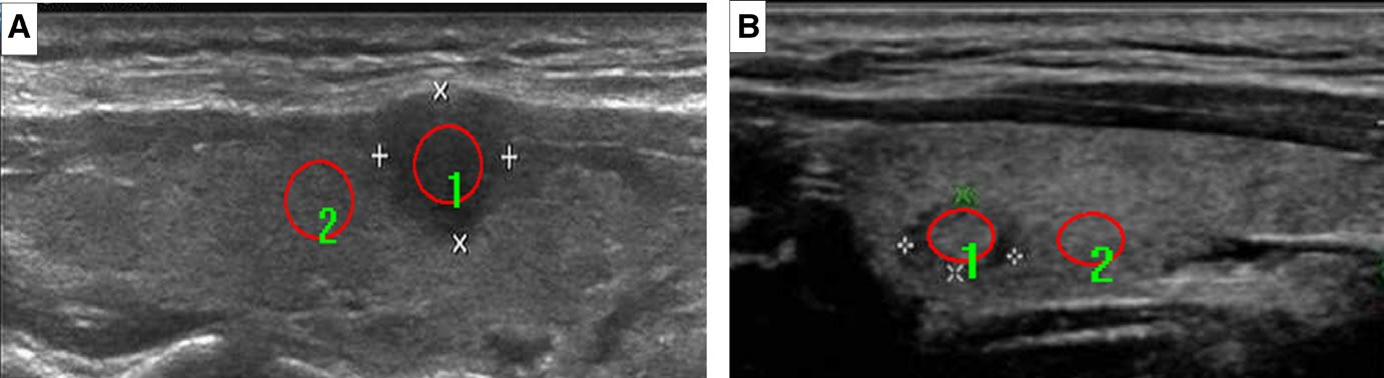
For PTCs with homogeneous echogenicity (A), regions of interest (ROIs) are drawn to include the nodule (1) and the surrounding normal thyroid tissue (2). The mean gray scale values for the PTCs and the surrounding normal thyroid tissue were 44.0 and 70.5, respectively, and the UGSR was 0.625 (44.0/70.5). For NGs with homogeneous echogenicity (B), the regions of interest (ROIs) are drawn to include the nodule (1) and the surrounding normal thyroid tissue (2). The mean gray scale values for the NGs and the surrounding normal thyroid tissue were 70.0 and 84.2, respectively, and the UGSR was 0.878 (70.0/84.2). Note that the ROI (red cycle) should be drawn around the largest area of homogeneous echogenicity nodules. PTCs, papillary thyroid carcinomas; NGs, nodular goiters; UGSR, ultrasound gray scale ratio
